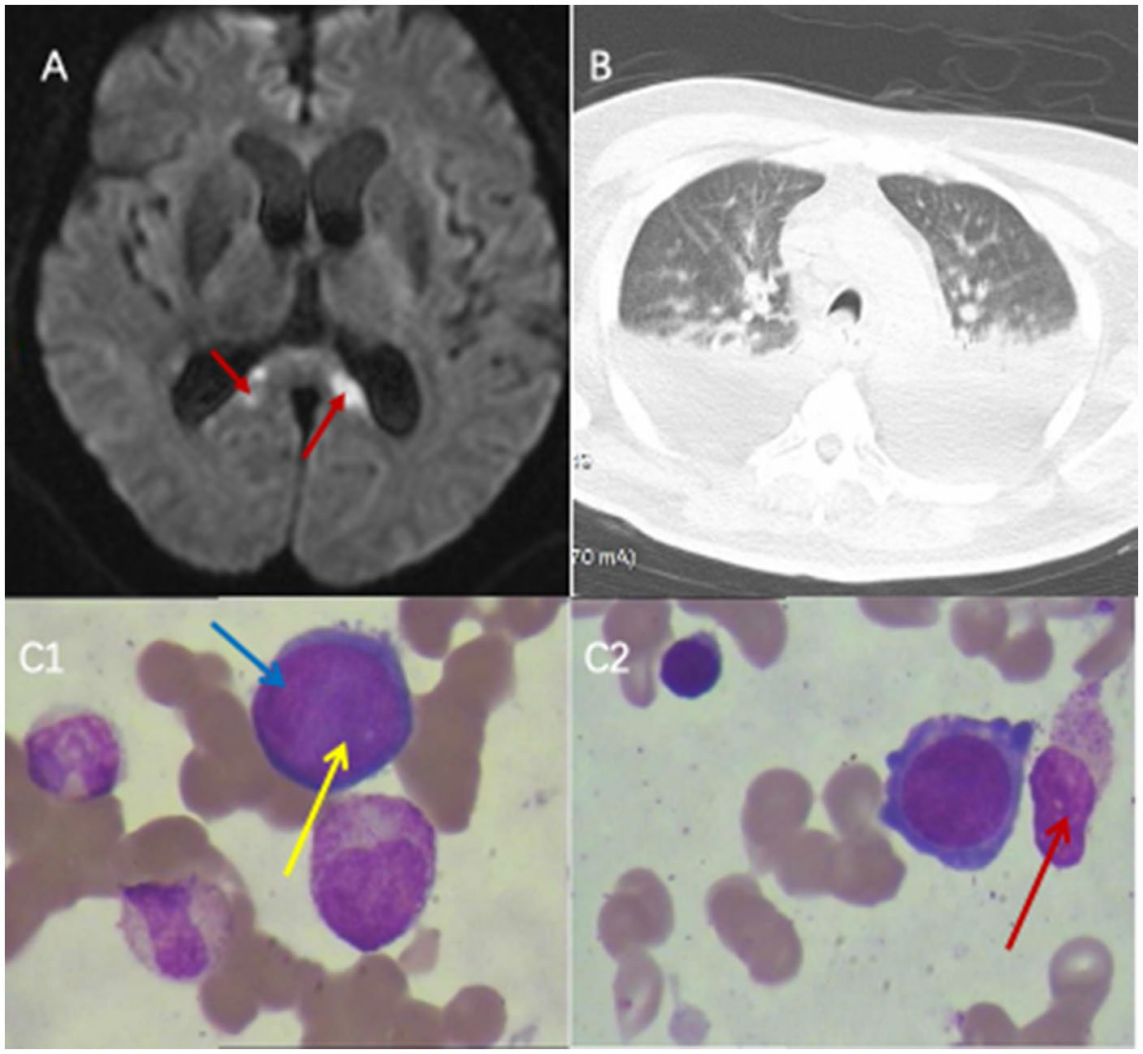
Results of head MRI, lung CT, and bone marrow puncture. (A) Brain magnetic resonance imaging (MRI) examination: Brain MRI revealed acute cerebral infarctions near the splenium of the corpus callosum and the posterior horns of the lateral ventricles. (B) Before being re-admitted to the ICU, the lung CT scan indicated bilateral pleural effusion. (C1, C2) Bone marrow smear of the patient. Proerythrocytes infected by parvovirus B19. Blue arrows indicated proerythroblasts, yellow arrows indicated viral inclusion bodies, red arrow indicates the plasma cells.
